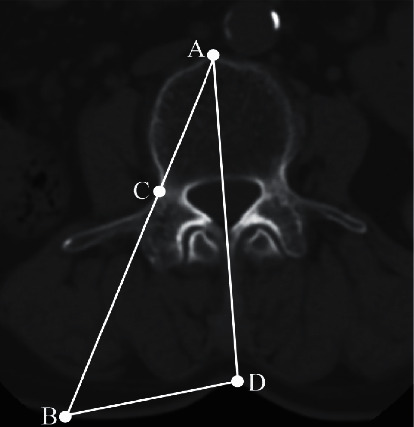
The skin entry point design for unilateral extrapedicular PVP. The skin entry point was determined from the axial image of preoperative CT at the target level. Point A is the junction point of the midline and the anterior edge of the vertebral body. Point D is the junction point of the midline and the skin. Point C is the entry point of the vertebral body. Point B is the junction point of the AC line and the skin, which is also the skin entry point of the unilateral extrapedicular PVP.
